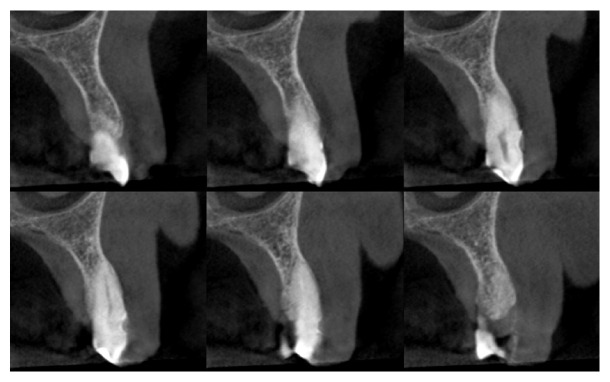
CT 10 months after biopsy.
